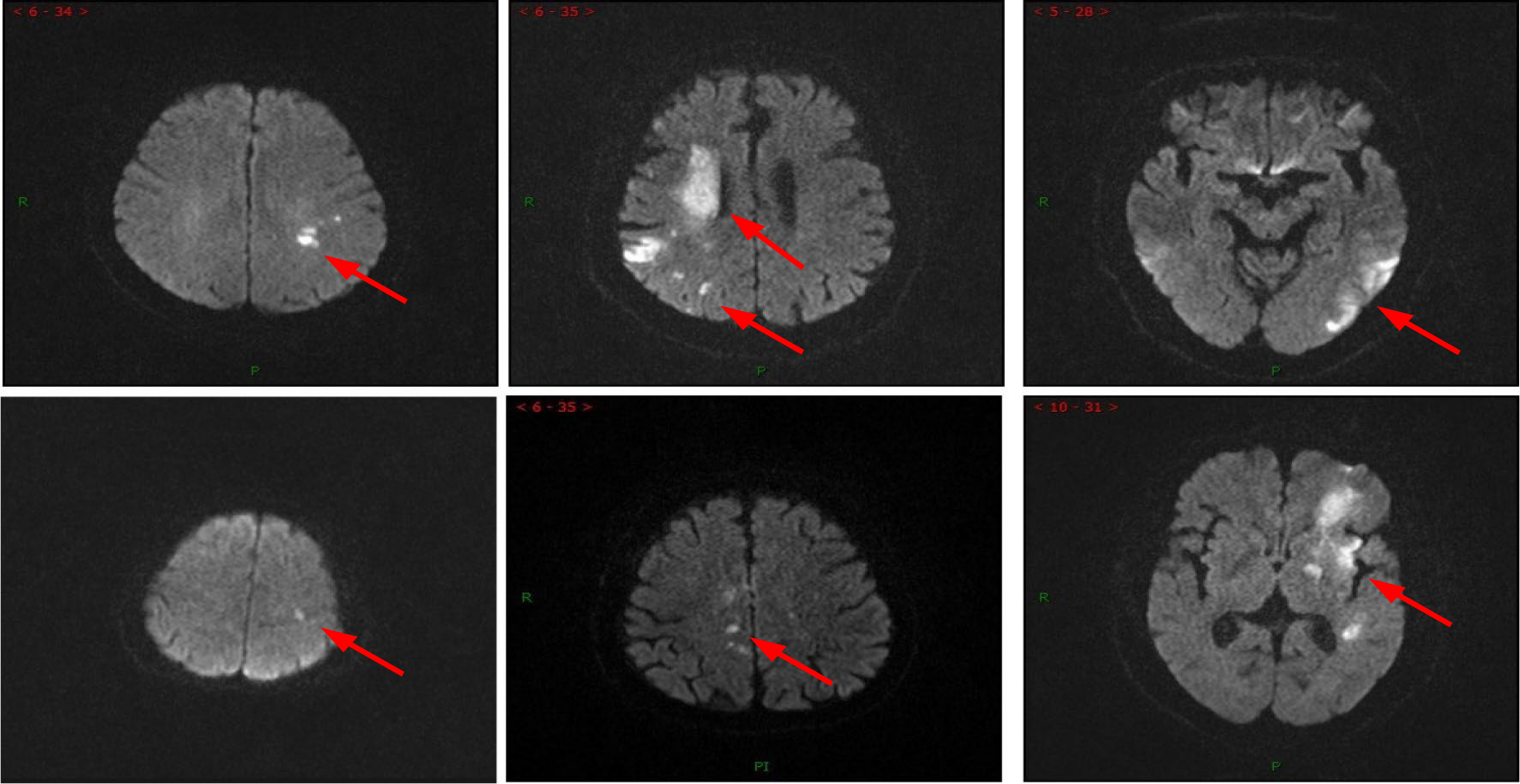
Example of the AIS patient MRI, where the red arrow indicates the focal area.
